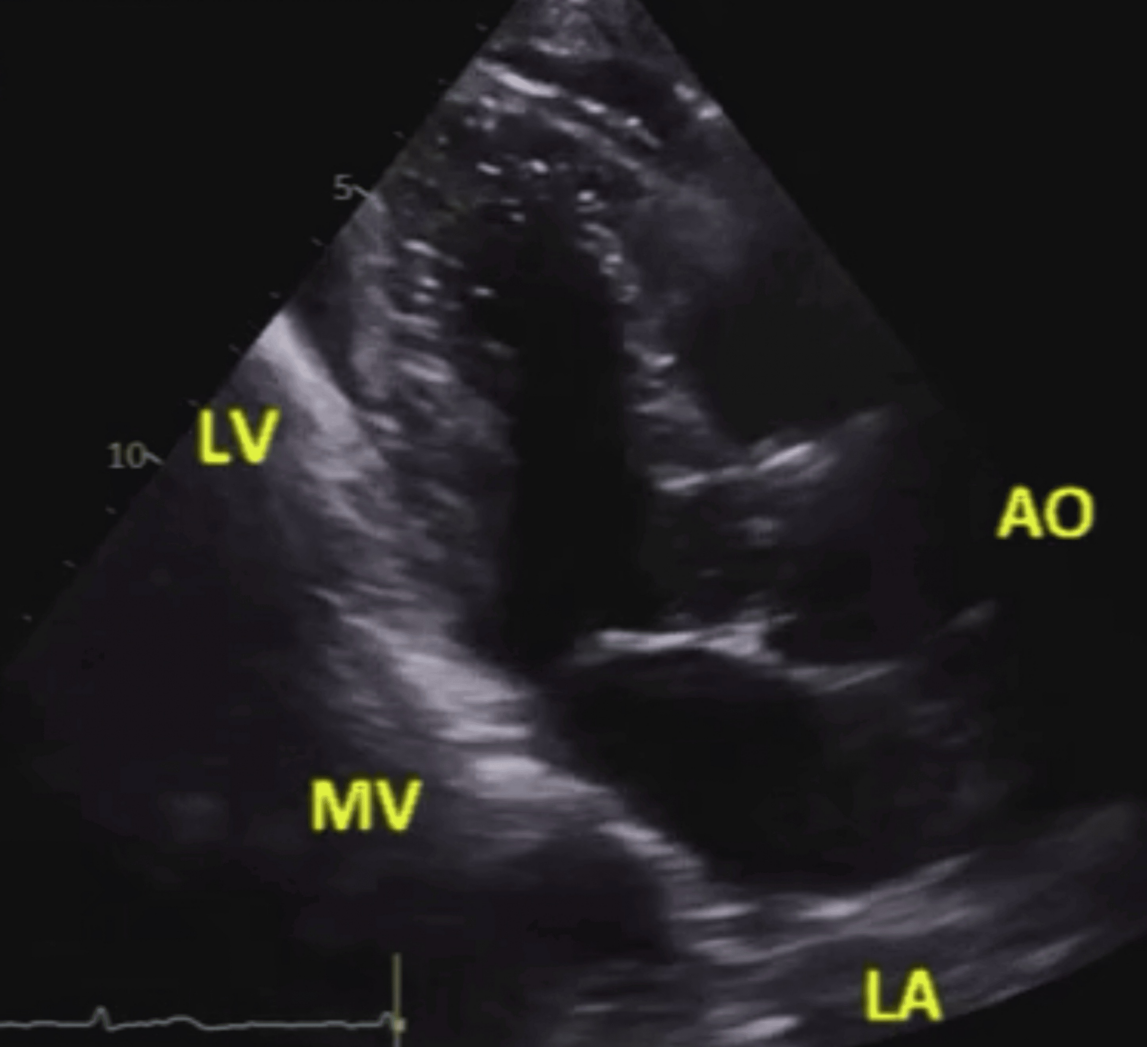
Two-dimensional echocardiography, parasternal long-axis view (off-axis), showing a static frame in systole Mitral annular disjunction and anterior mitral valve prolapse are not clearly recognized. LV: left ventricle; LA: left atrium; MV: mitral valve; AO: aorta
